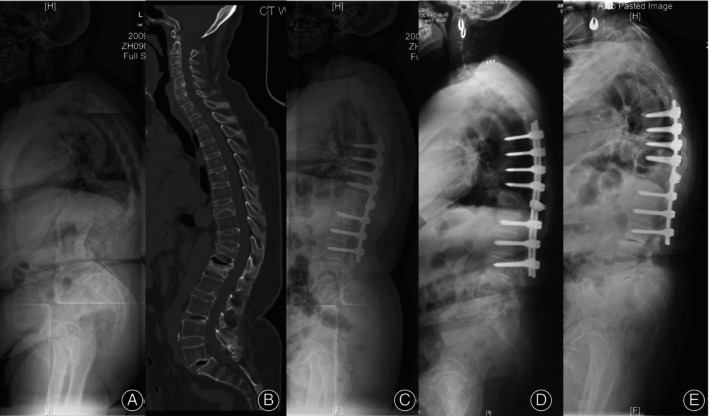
A 65‐year‐old woman presented with severe back pain. (A). The standing long‐cassette lateral radiographs showed thoracolumbar hyper‐kyphosis arising from osteoporotic vertebral compression fracture (OVCF) and L5 spondylolisthesis, global kyphosis (GK) was 82.3°and thoracolumbar kyphosis (TLK) was 53.4°, sagittal stable vertebrae (SSV) located at L3. (B) Computed tomography revealed T9, T11, T12, and L1 fractures. (C) Postoperative lateral x‐ray showed T7‐L3 fixation and T11‐12 modified grade 4 osteotomy, GK decreased to 32.5°and TLK decreased to 6.2°, SSV moved cranially to L2. (D) Wedging in L34 disc was detected at 3‐years' follow‐up. (E) Degenerative changes progressed in L34 disc at 7‐years' follow‐up, GK and TLK was 55.1° and 10.6°, respectively.
